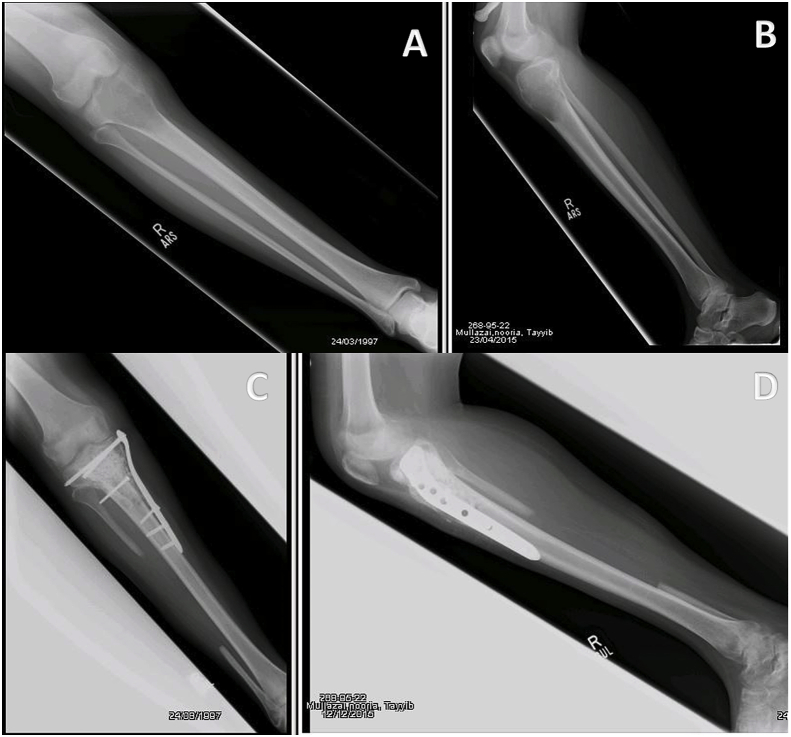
(A & B): Preoperative x-rays of 18 years old girl with knee pain for 4 months showing the typical appearance of GCT in proximal tibia along with soft tissue extension. (C & D): Postoperative x-rays after extended curettage, fibula bone graft, PMMA and stabilization of locking plate.
